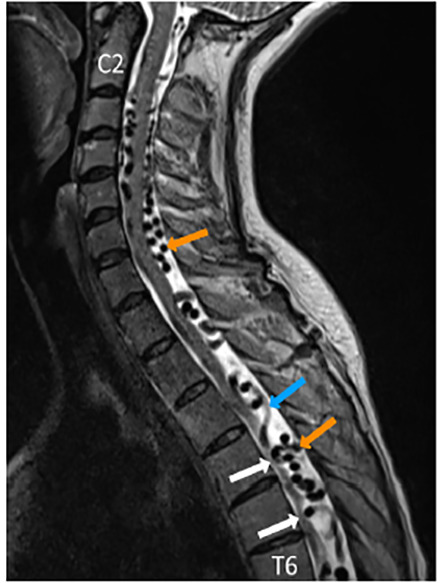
Sagittal magnetic resonance imaging T2-weighted image of the cervical and thoracic spine. Flow voids appear black against white cerebrospinal fluid, and indicate extensive dilated vessels suggestive of dural arteriovenous fistulas. Orange arrows identify vessels perpendicular to the plane of the image, blue arrow identifies vessel at an angle to the plane with relatively increased signal. The white arrows highlight areas of spinal cord being compressed or deformed out of plane by dilated vessels.
